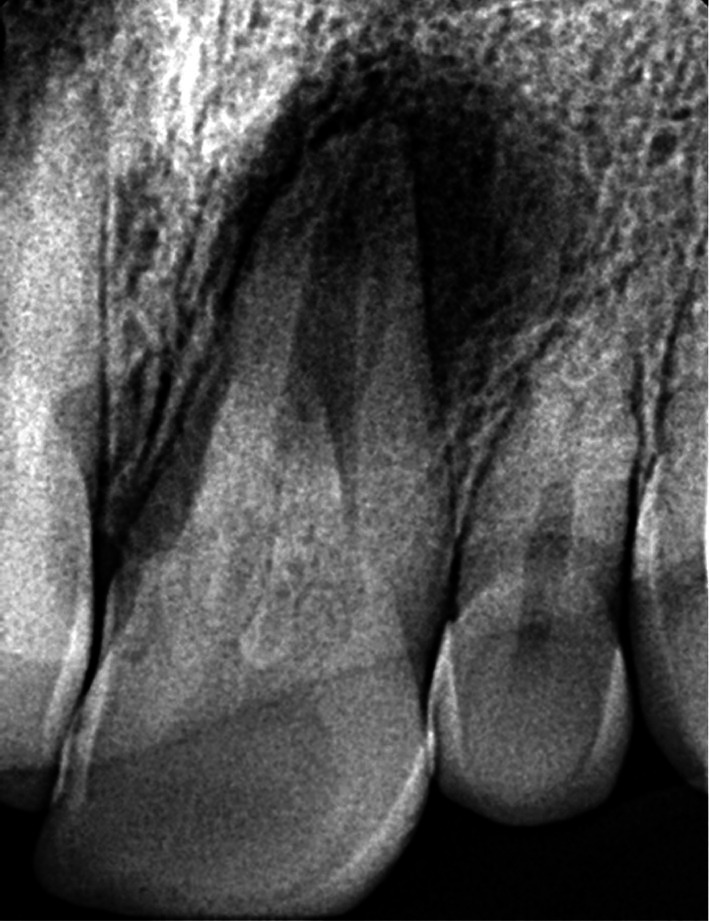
Initial radiographic view.
